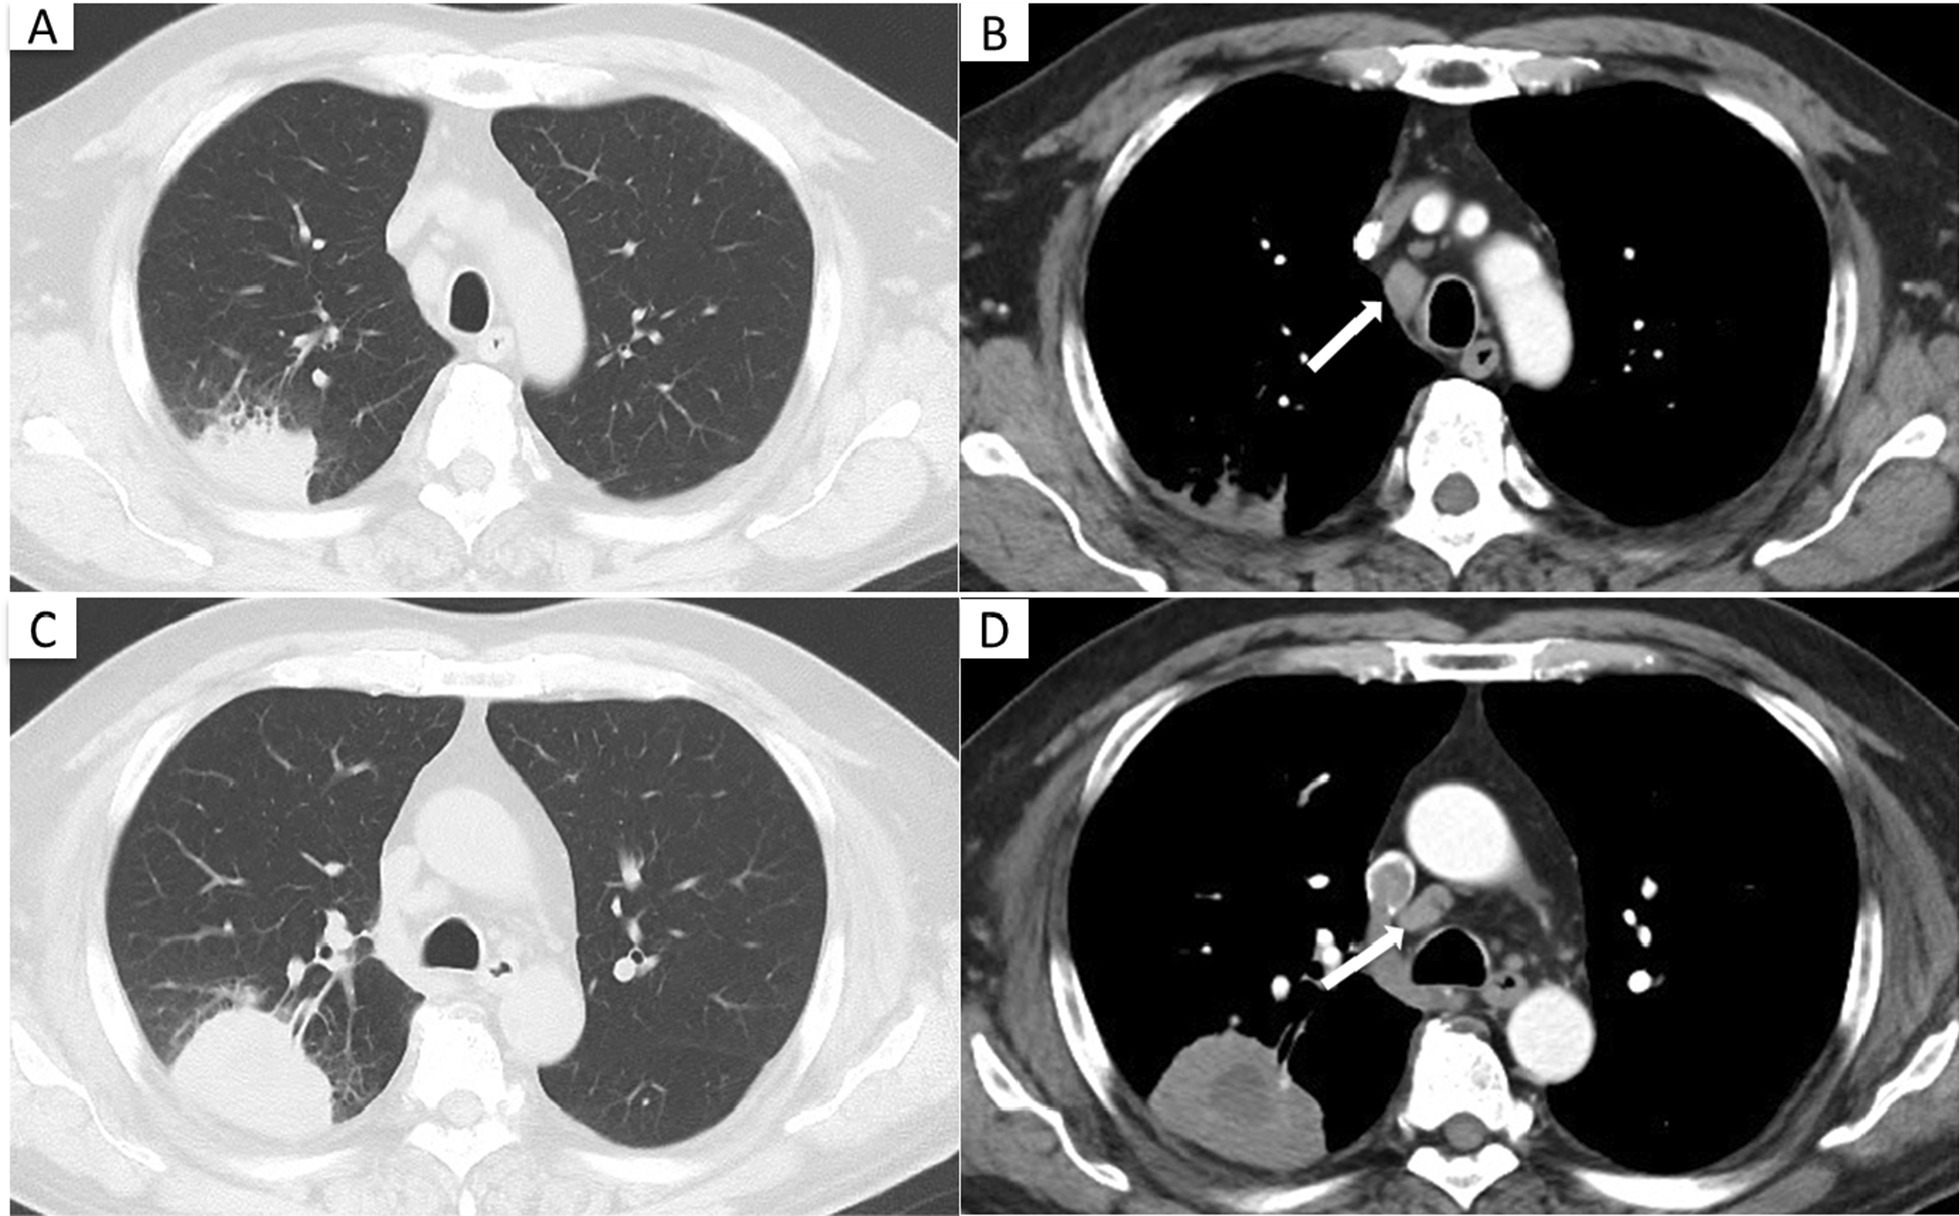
Chest CT on admission. Contrast-enhanced CT showed an irregularly shaped nodule in the upper lobe of the right lung, mediastinal lymphadenopathy (arrow), and hilar lymphadenopathy (A, C lung windows; B, D soft-tissue windows)
